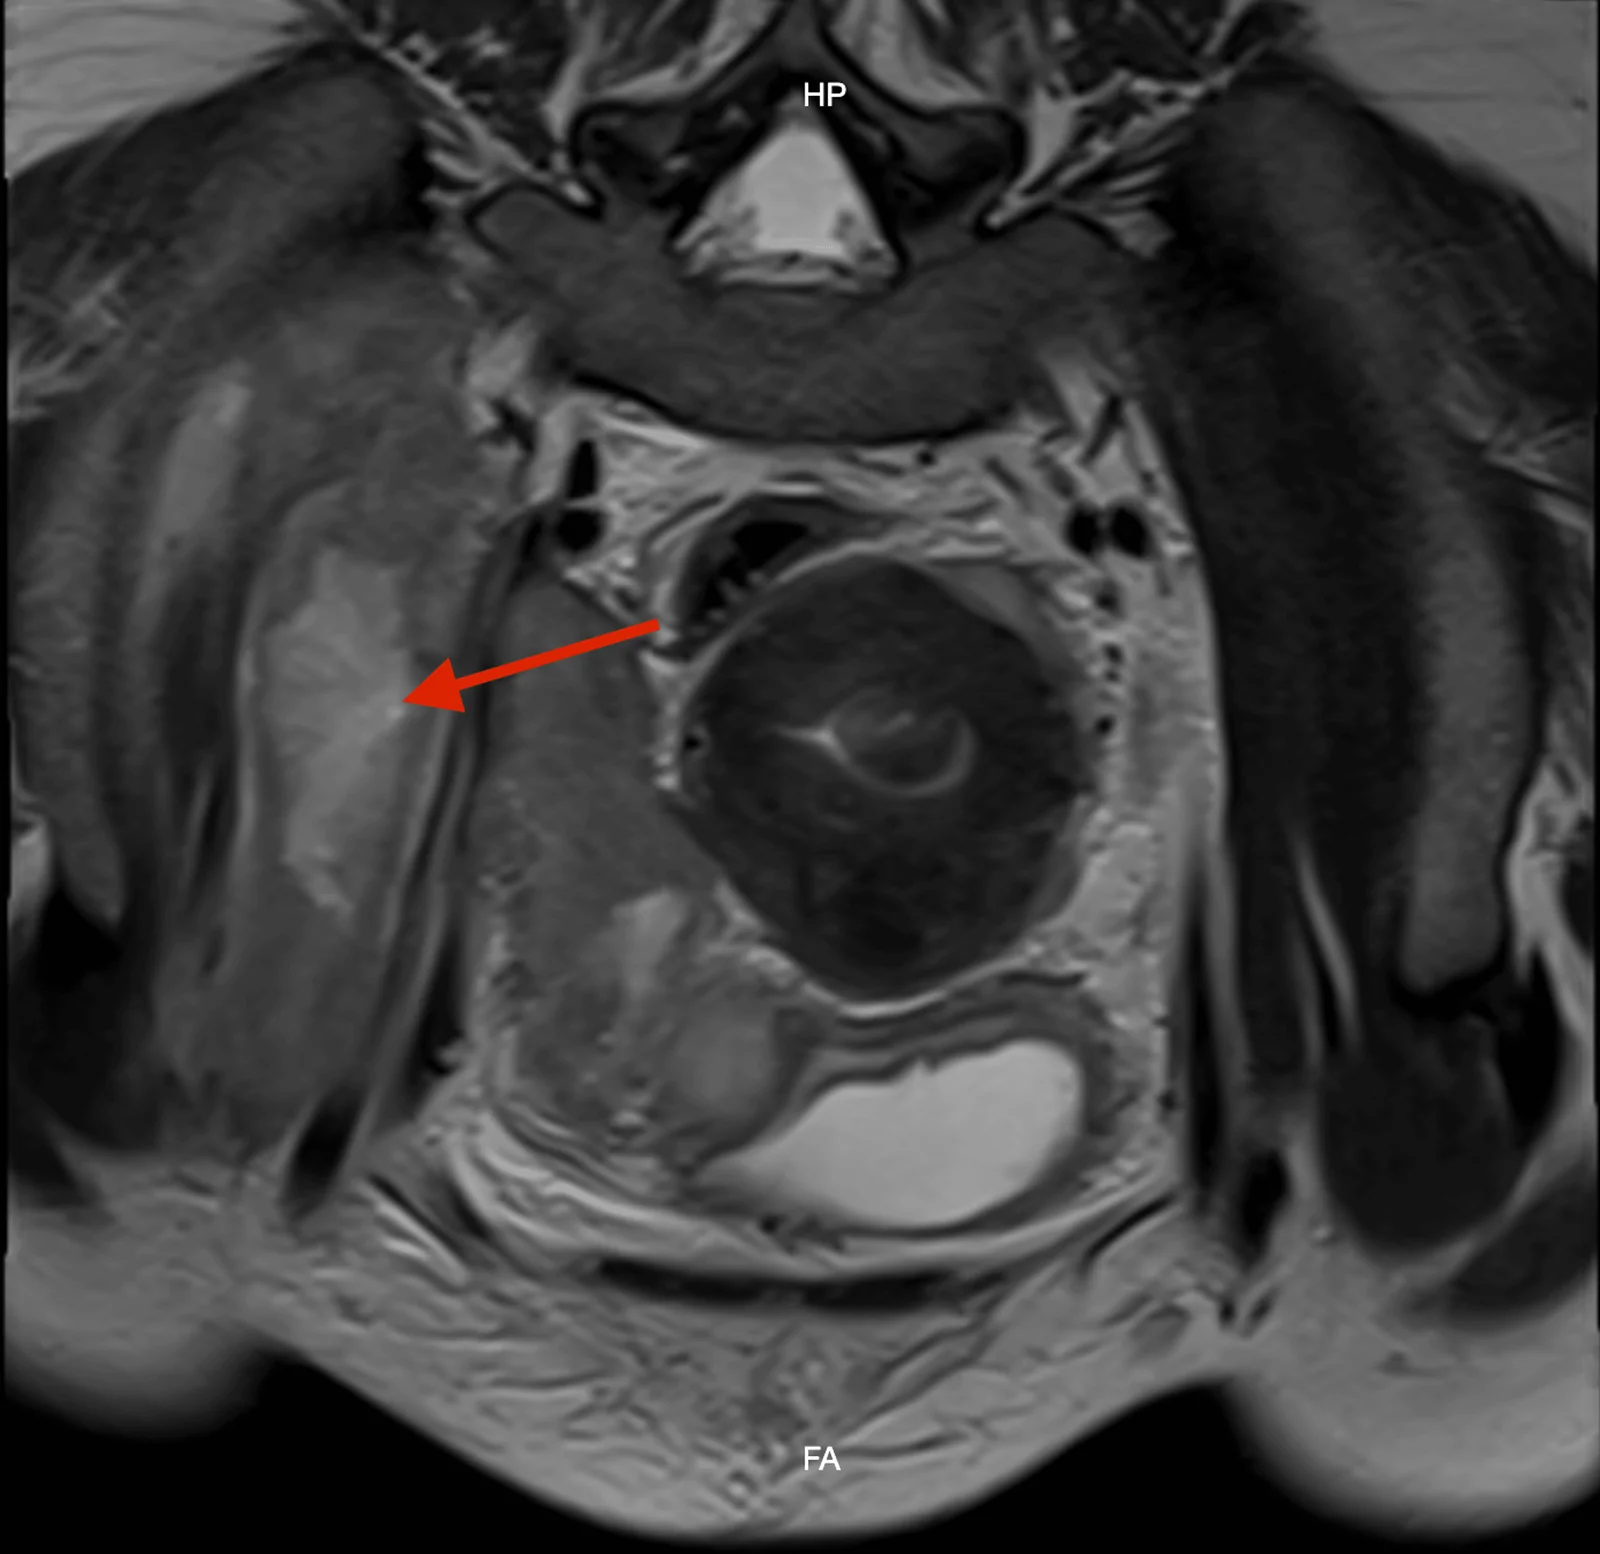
Magnetic resonance imaging of the abdomen and pelvis in the coronal plane, T2-weighted sequence. Focal solid lesion in the right iliopsoas muscle, indicated by the arrow. HP: head-posterior; FA: foot-anterior
